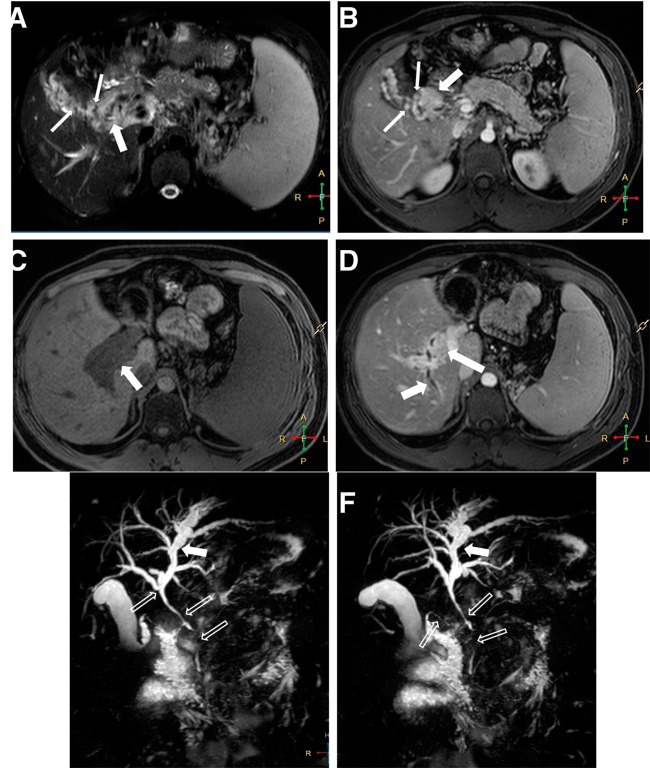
Focal and circumferential wall thickening with enhancing foci, suggesting epicholedochal veins and fibrosis (thick arrows), gallbladder varices (thin arrows) appear as low signal intensity on fat saturated T2-weighted imaging (A) and as enhancing on contrast-enhanced fat-saturated T1-weighted image (B). Biliary ductal wall thickening indicates hypointensity on fat-saturated T1-weighted imaging (C) and enhancement on fat-saturated imaging (B, D) (thick arrows). MRCP images (E, F) show multiple biliary stenoses involving the common bile duct (CBD) and proximal right hepatic duct are seen with proximal bile duct dilation (open arrows). Axial fat-saturated T2-weighted imaging (A), axial contrast-enhanced fat saturated T1-weighted imaging (B) at the same level with (A), axial fat-saturated T1-weighted (C), axial contrast-enhanced fat saturated T1-weighted imaging (D) at the same level with the (C), and MRCP images (E and F).
